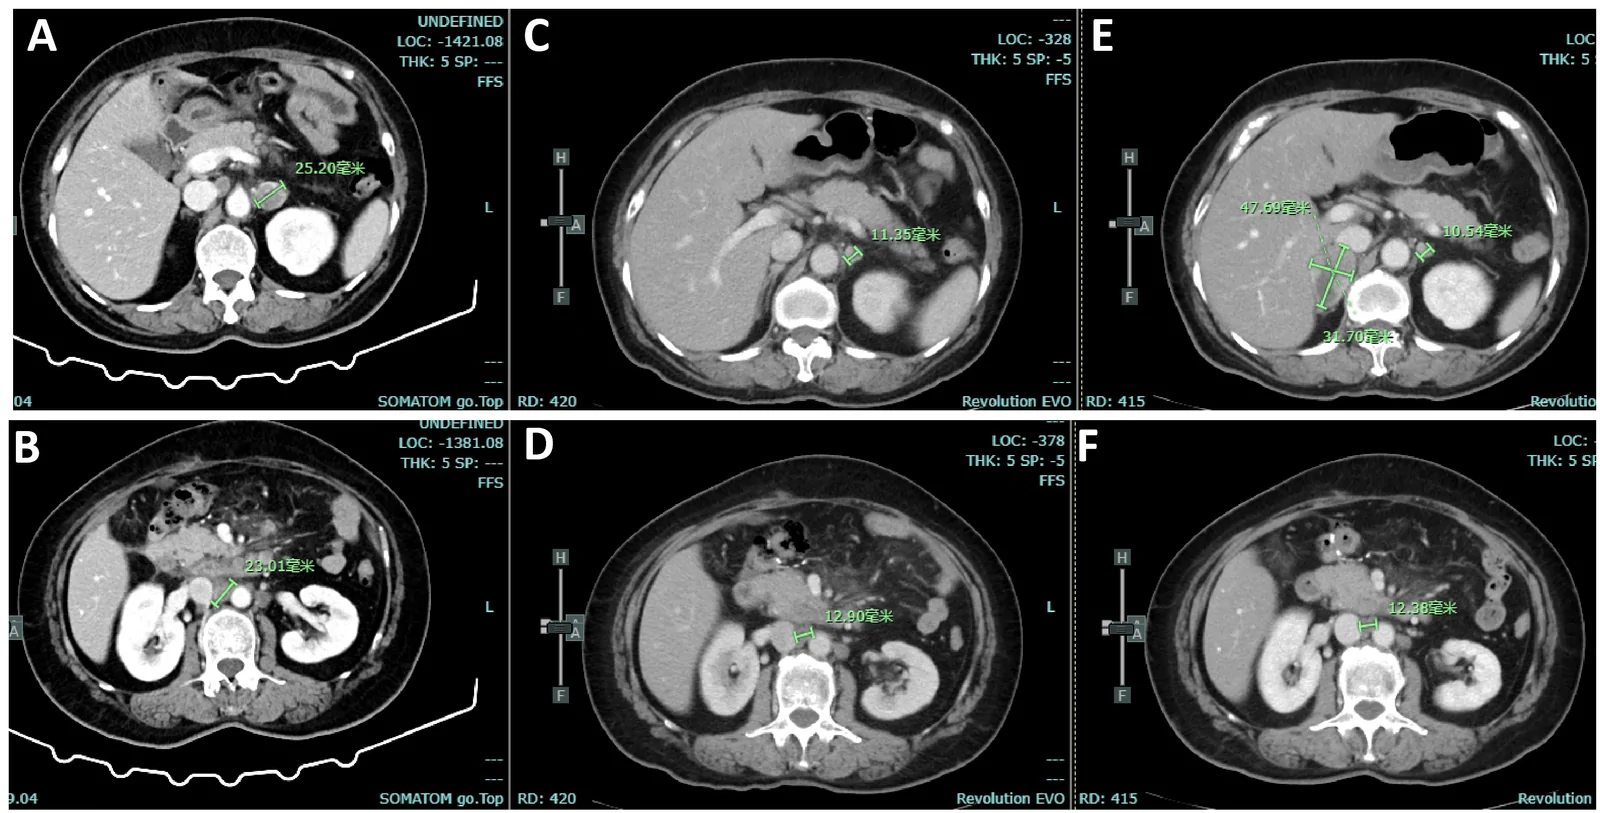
Serial CT images during fifth-line sintilimab plus chidamide therapy. (A, D) Baseline (February 21, 2024). Sum of target lesions: 4.8 cm. (B, E) Best response (May 27, 2024). Sum of target lesions reduced to 2.3 cm (−52.1%); per RECIST, best overall response: partial response (PR). (C, F) Progression (August 26, 2024). Target lesions stable, with new right adrenal lesion; per RECIST, progressive disease (PD). Scan dates are shown in each panel. All images are at comparable anatomical levels.
